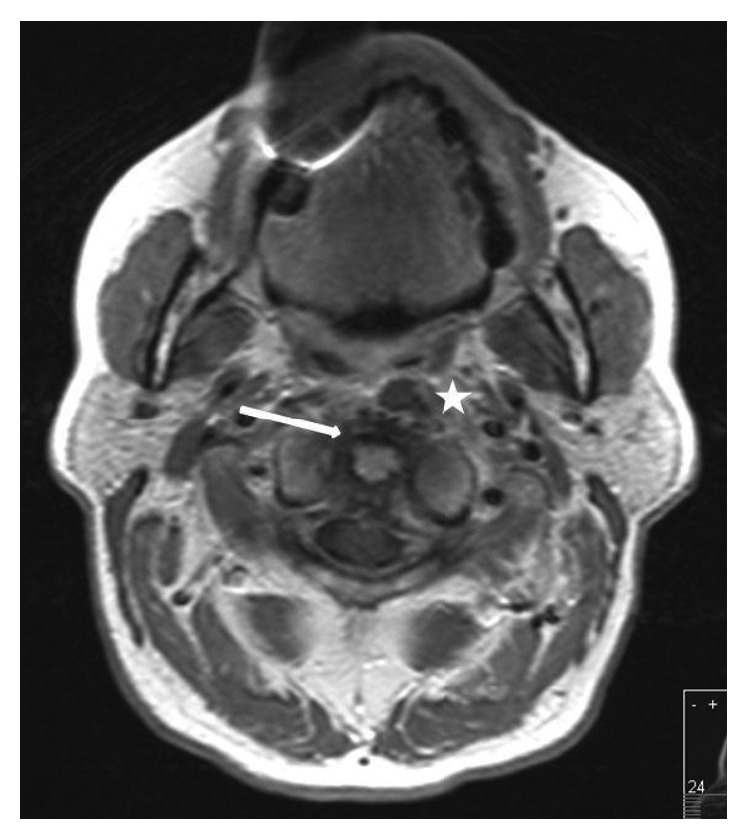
Axial MRI slice at initial presentation: contrast-enhanced T1-weighted axial MRI scan at the level of the dens axis. The white arrow identifies an annular enhancement round the dens axis while the white asterisk indicates the focus ventromedial of the left arch of the Atlas with 11 × 8 mm in diameter.
